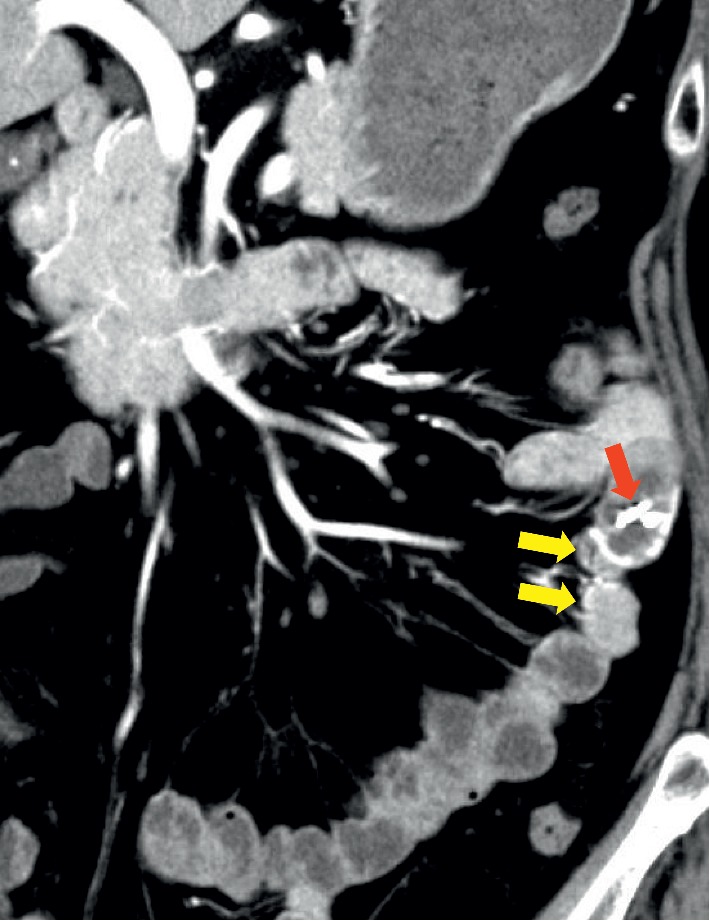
Multiple-phase computed tomography. Small vascular ectasias (yellow arrows) during the arterial phase were observed adjacent to the clips (red arrow).
